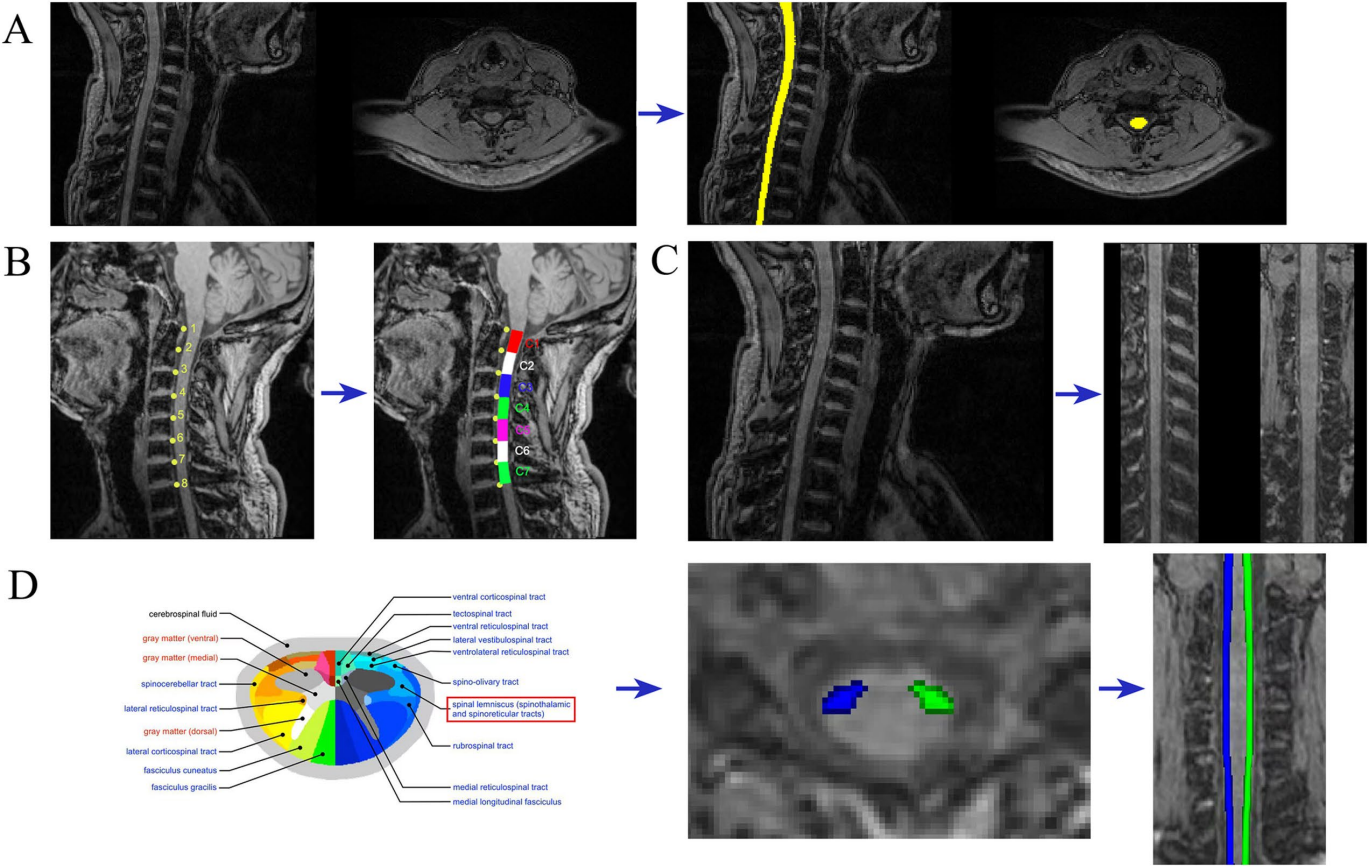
Imaging analysis. Examples of MRI metric analysis showing (A) spinal cord segmentation; (B) spinal cord segmental delineation; (C) spinal cord “straightened” (D) spinothalamic tract segmentation.
